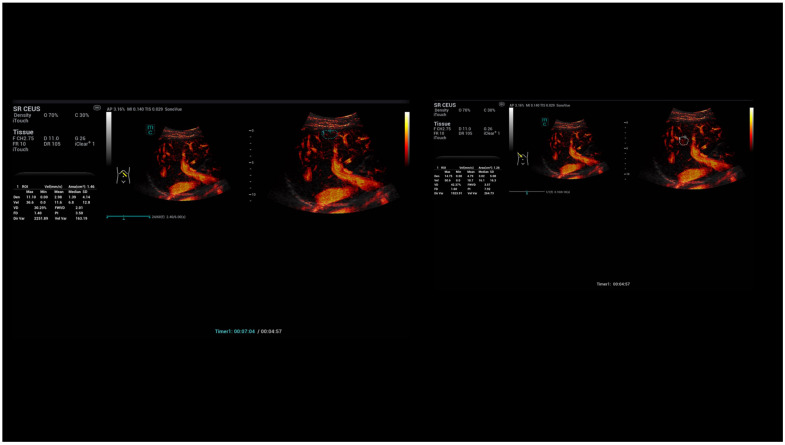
Area measurements of capillary vascalary structures near the liver capsular structure and in the center of the liver using a integradet SR CEUS quantification software. Independent evaluation by the stored dynamic SR CEUS cine loops over 5 s with manually adapted area’s near the capsular structures in comparison to the centre with peripheral capillary dilation in a case of Osler’s disease. CEUS in arterial phase after bolus injection of 2.0 mL ultrasound contrast agent (SonoVue) using a convex multifrequency probe (1–7 MHz).
